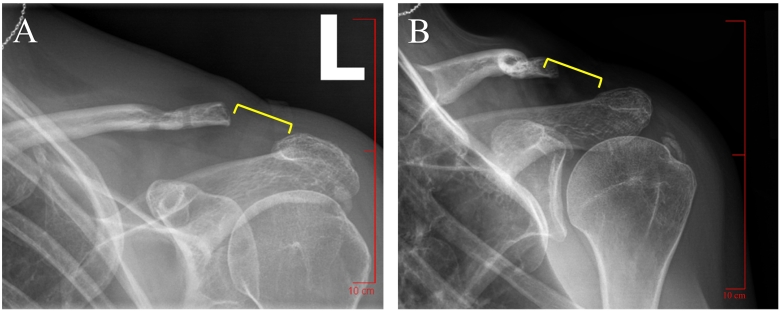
Anteroposterior (image A) and grashey (image B) radiographs demonstrating distal left clavicular erosion (yellow bracket) prior to second revision surgery for graft material removal, subacromial decompression, calcium debridement, and arthroscopy. (For interpretation of the references to colour in this figure legend, the reader is referred to the web version of this article.)
